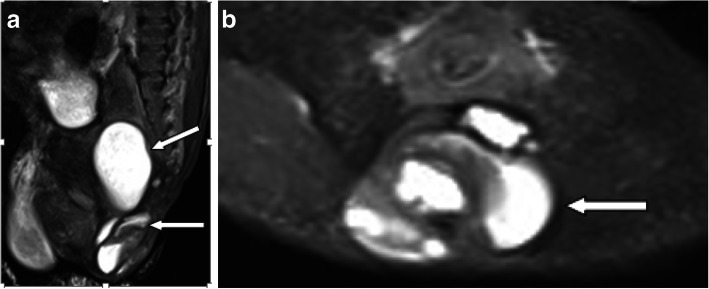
aMRI sagittal T2W1 image of the SCT at two days of age. b MRI axial T2W1 image of the SCT at two days of age
